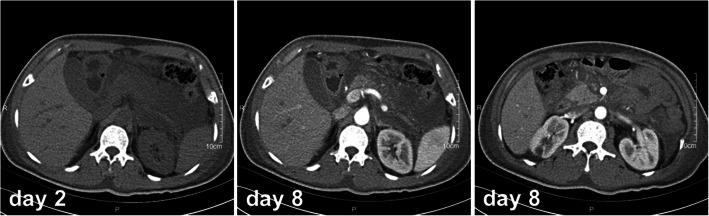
Abdominal computed tomography exhibited acute necrotizing pancreatitis with peripancreatic effusion and local intestinal wall thickening on day 2 and day 8 of admission
